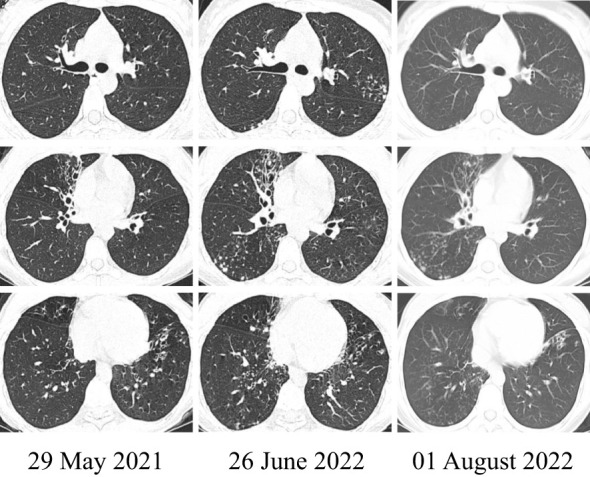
The representative image: changing in the patient’s chest CT. The CT on June 26, 2022, compared with May 29, 2021, showed more serious pneumonia with bronchiectasis in the RML and LLL. However, after the identification of *Nocardia* species and systemic treatment, the imaging on August 1, 2022, showed improvement.
